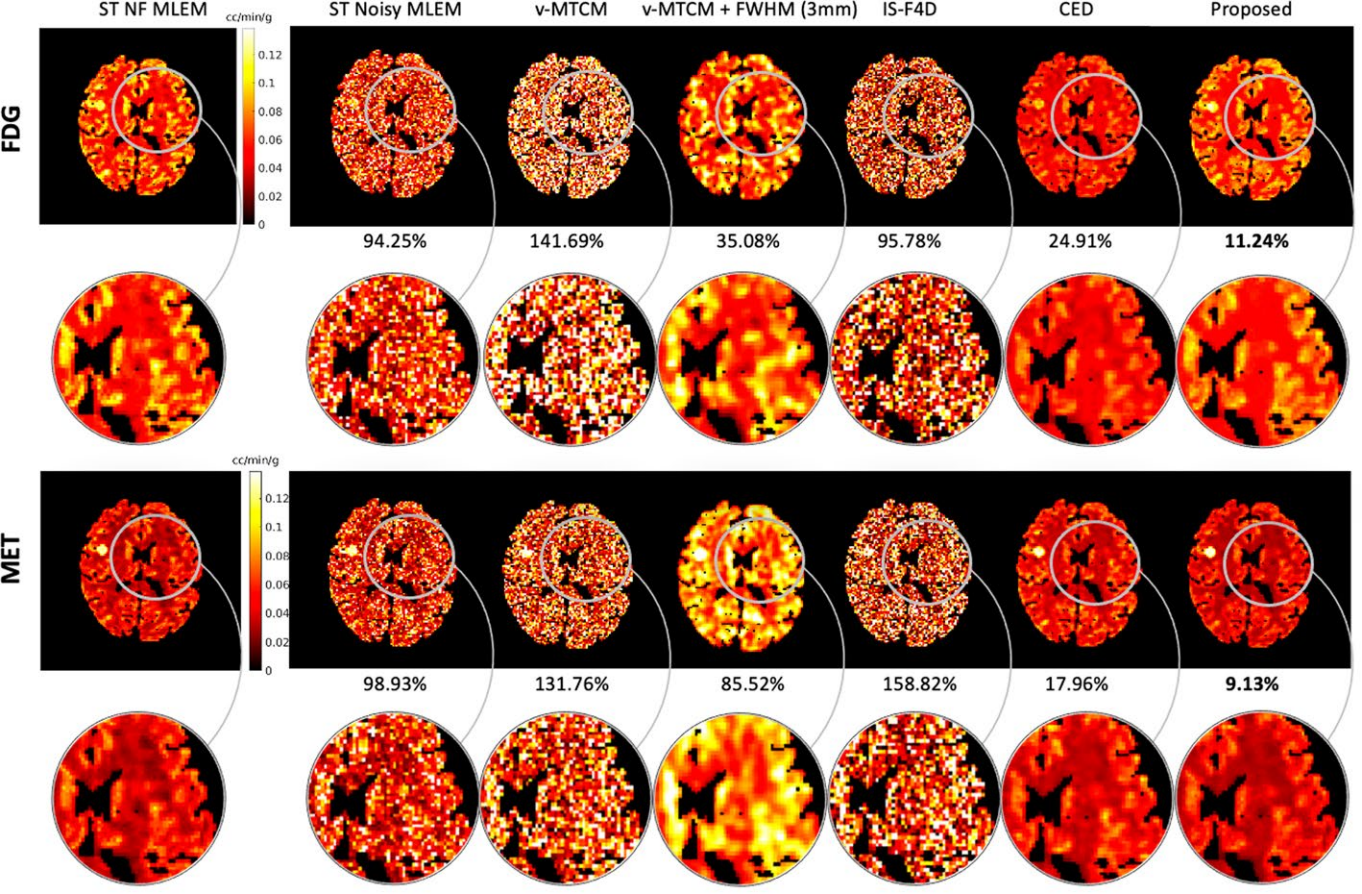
A test example of the parametric maps $$K_1$$ estimated from the separated single-tracer activity images for FDG and MET, respectively (also see Fig. 3 for the ground-truth $$K_1$$ images). NRMSE values of each estimated $$K_1$$ image were shown at the bottom. For plotting only, we limit the colour scale of the figures to fall within the same range as the reference images shown in the first column
